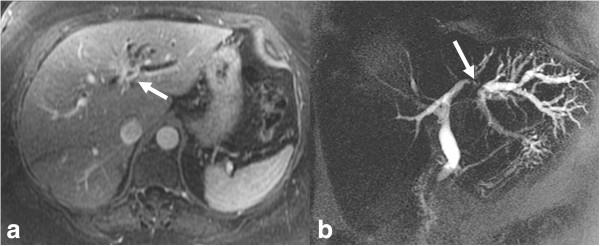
Infiltrating pCCA. Axial post contrast enhanced T1-weighted MR image (a) and MRCP (b) images demonstrating an enhancing stricture involving the left hepatic duct (arrow) with upstream dilatation of the left hepatic ducts.
